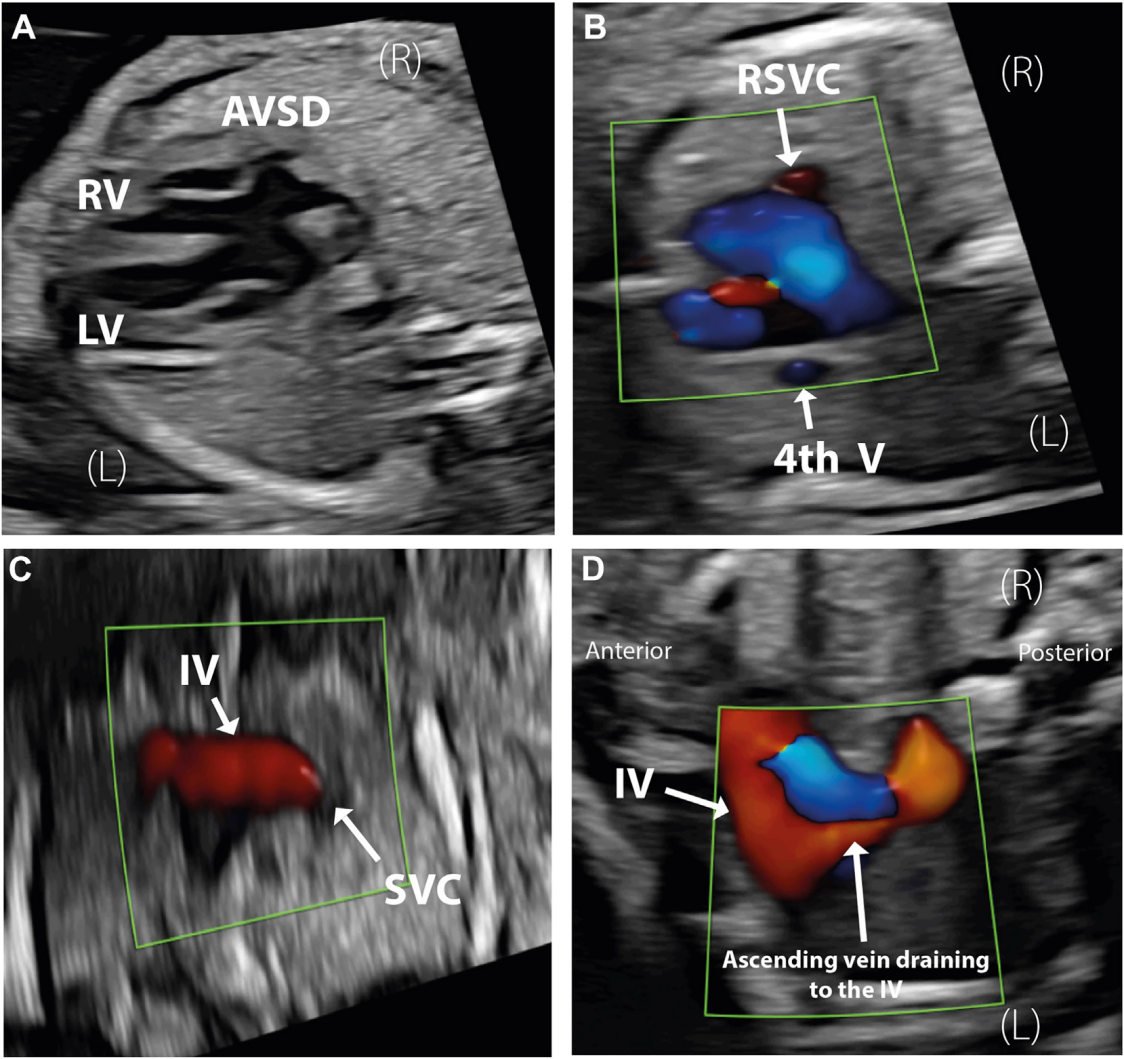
Two-dimensional fetal echocardiogram, obtained at 21 weeks of gestation, transverse plane, four-chamber view (A), demonstrates levocardia, two balanced ventricles, and a common atrioventricular valve consistent with an AVSD; transverse plane, three-vessel view superior sweep with color flow Doppler (B) demonstrates four vessels, different color displays of the two venous vessels (opposite direction of flow), a prominent IV (C) with an ascending vein to the left of the PA draining to the IV (D). *4th V*, Fourth vessel; *LV*, left ventricle; *RV*, right ventricle; *SVC*, superior vena cava.
